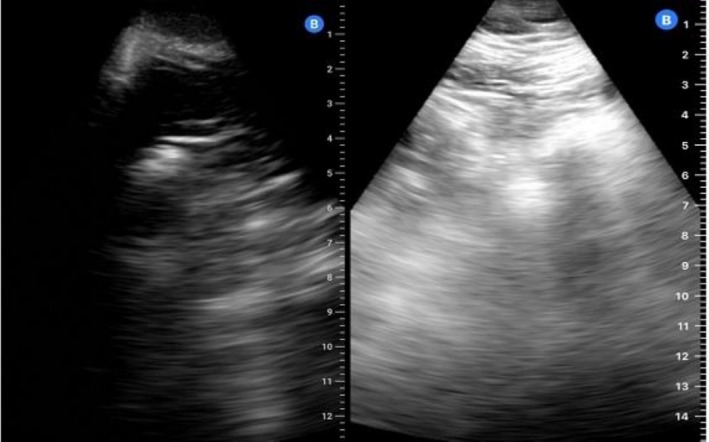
Patient 1. Ultrasound performed with the portable “Butterfly” device of the left lung base in posterior view, showing a small subpleural and intraparenchymal infiltrate (left lateral on the left) and an increase in consolidation foci and loss of the A‐line pattern (left medial on the right).
